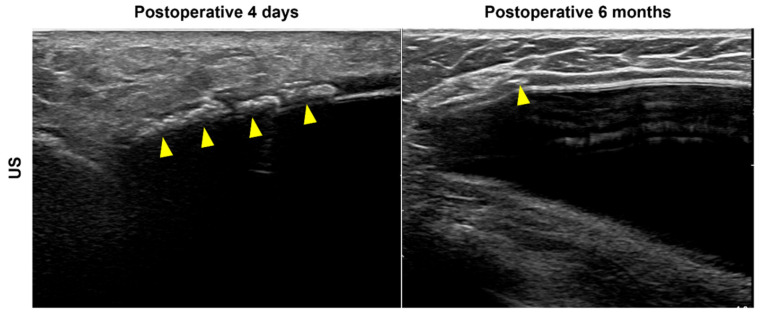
Acellular dermal matrix (ADM) ultrasound (US) findings in implant-based breast reconstruction. US imaging immediately after surgery (postoperative day 4) shows bright, hyperechoic dots diffusely visible in the ADM (arrowheads, left). After six months, the number of bright echogenic spots in the ADM reduced considerably (arrowhead, right).
